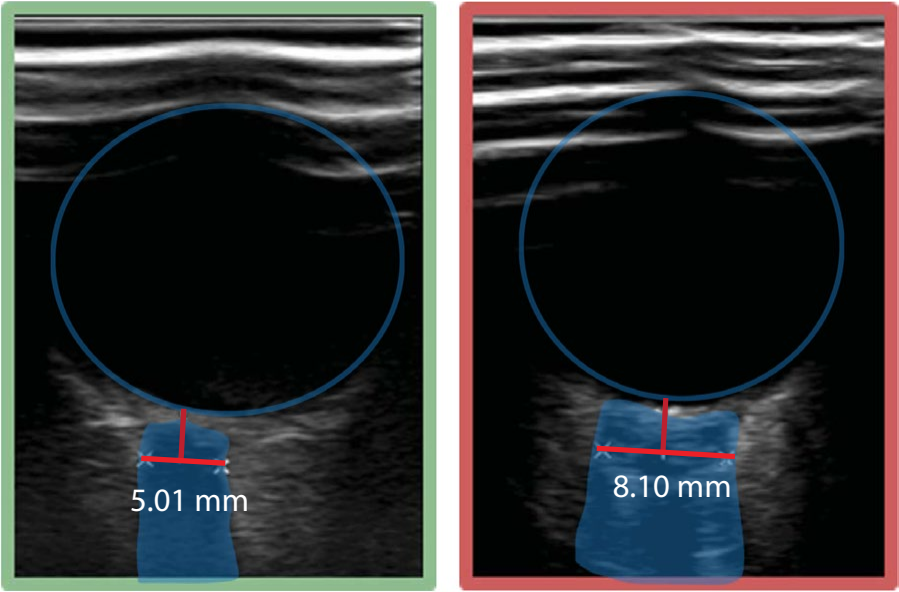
Examples of ultrasound images of the ONSD for a patient with good neurological outcome (left, ONSD = 5.01 mm) and a patient with poor neurological outcome (right, ONSD = 8.10 mm). The eyeball and optic nerve including its sheath are delineated in blue. The red horizontal line indicates the ONSD
